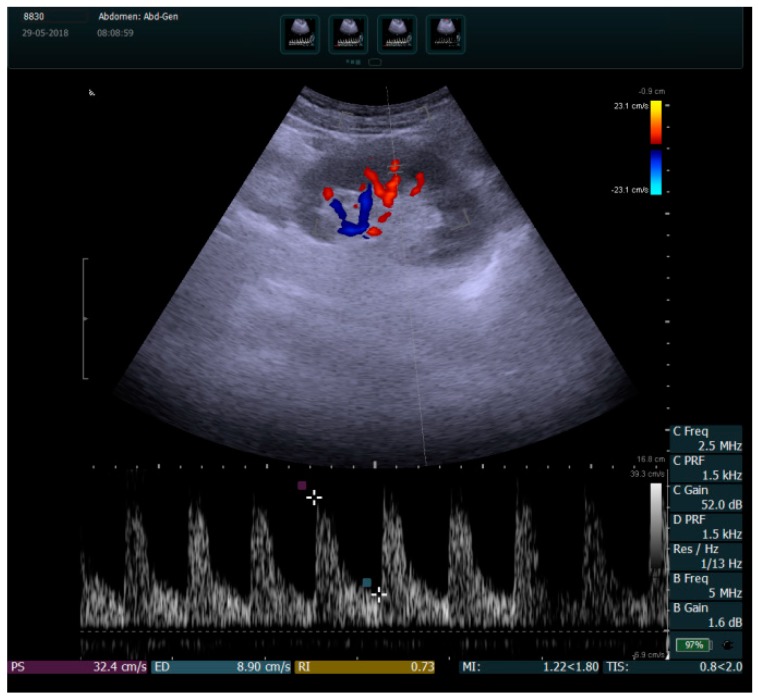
Color duplex sonography of the left kidney intrarenal segmental arteries. In this case, the renal resistive index (RRI) interval is 0.73, measured in a patient with ischemic heart failure.
